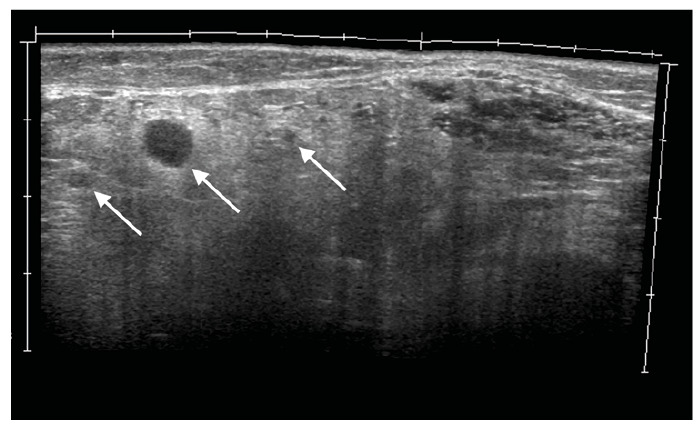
Ultrasound of the subcutaneous layer of the forearm displaying several granulomas (arrows).
